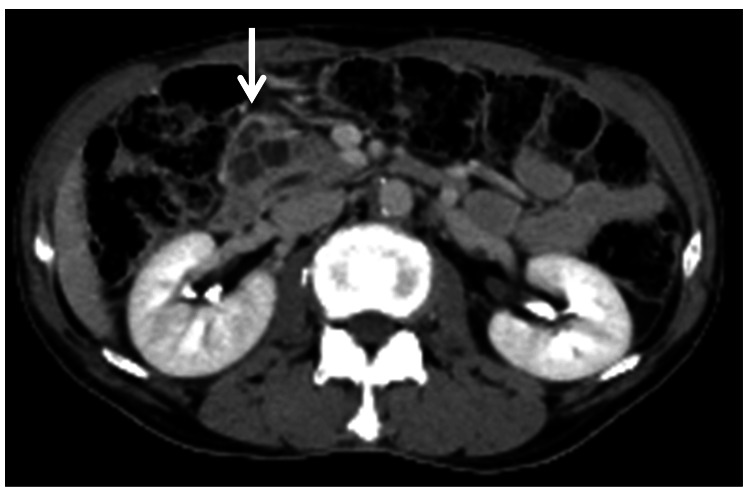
Contrast-enhanced CT revealing a polycystic mass with a diameter of about 40 mm
